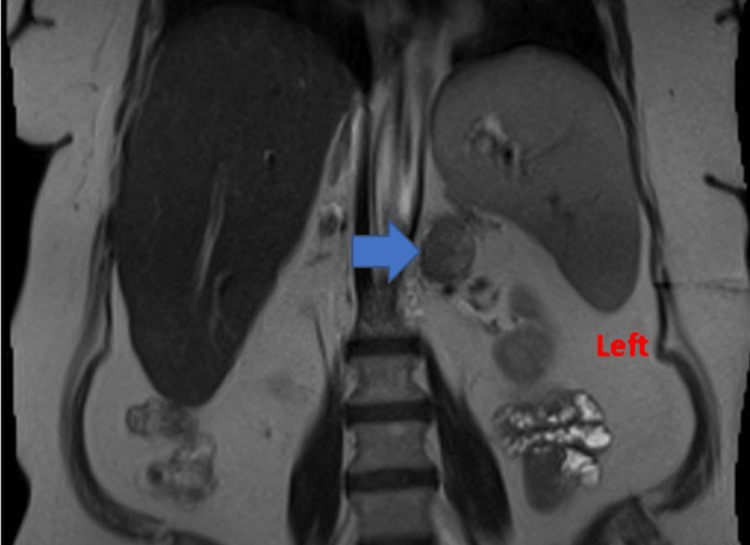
Magnetic resonance imaging of the abdomen depicting the left adrenal mass (blue arrow)
